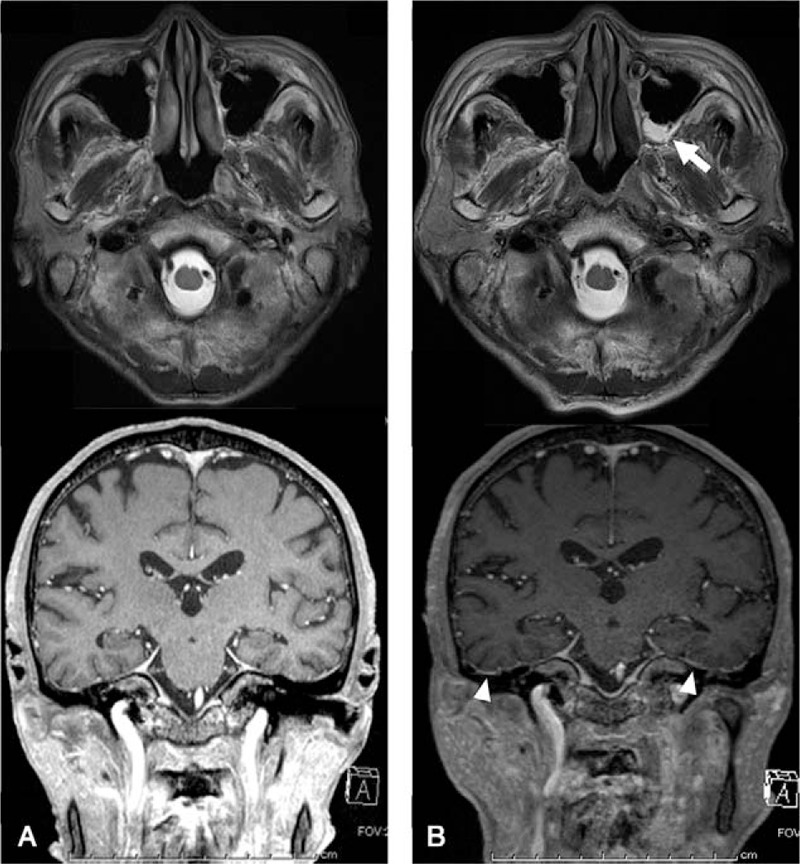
Magnetic resonance imaging of the head. (A) One month after the initiation of remission induction therapy. (B) At the time of relapse. The white arrow indicates the fluid in the left maxillary sinus, and the white arrowheads denote gadolinium enhancement of the hypertrophic dura mater.
